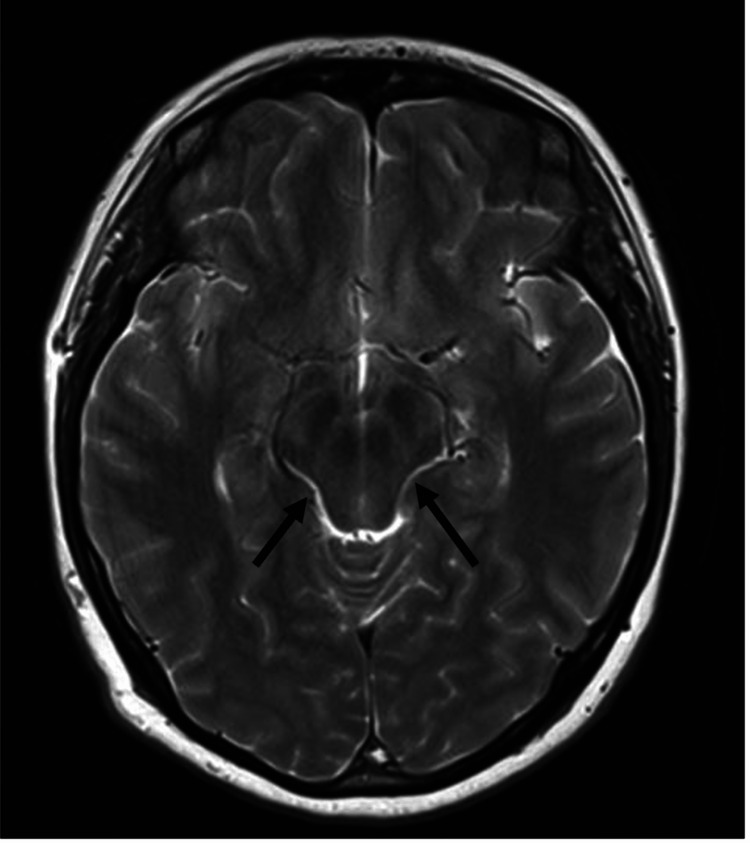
Brain MRI, axial T2, showing improvement in the effacement of the basal ambient cisterns (arrows)
